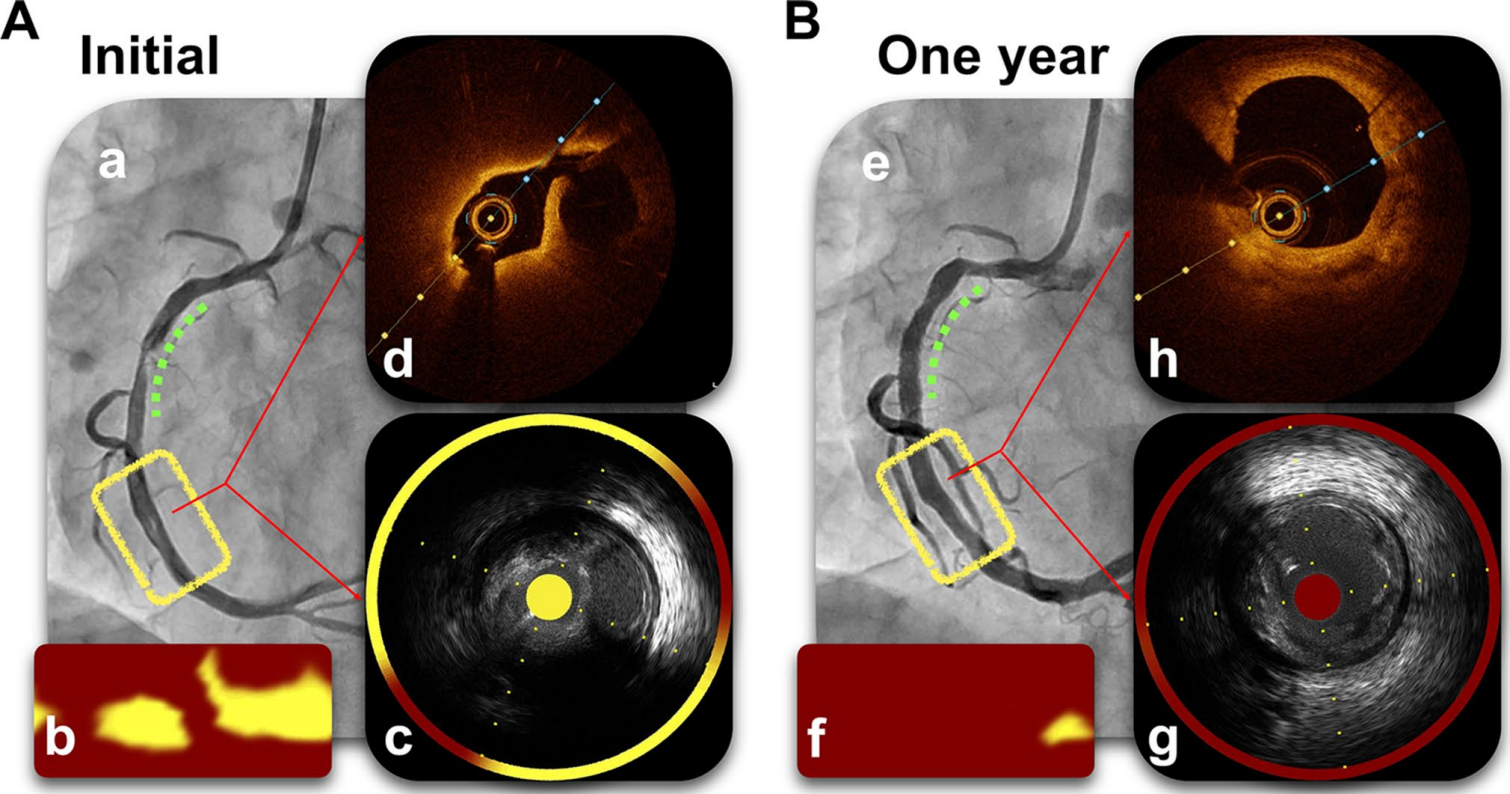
Drastic changes in the coronary images of a ruptured non-culprit lesion. A Initial coronary images: a coronary angiography, b and c initial NIRS-IVUS images, and d Initial OCT image of the non-culprit lesion. B One-year follow-up coronary images: e follow-up coronary angiography, f and g follow-up NIRS-IVUS images, and h follow-up OCT image of the non-culprit lesion. Green dotted lines denote the culprit lesions; yellow lines denote the non-culprit lesions. The maxLCBI_4mm_ in the non-culprit lesion has significantly decreased in the follow-up NIRS-IVUS analysis (from 743 to 126) (b, f). The disrupted fibrous cap in the non-culprit lesion has disappeared in the follow-up OCT analysis (d, h). *maxLCBI*_*4mm*_ maximum 4-mm lipid core burden index, *NIRS-IVUS* near-infrared spectroscopy intravascular ultrasound, *OCT* optical coherence tomography
